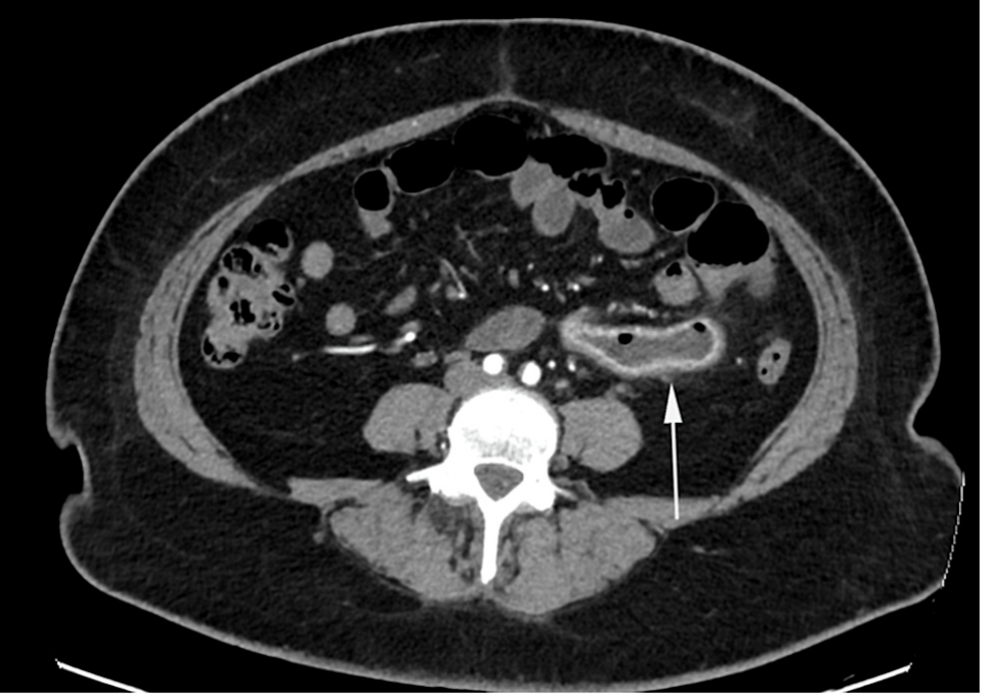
Axial reformatted, post intravenous contrast computed tomography images in the arterial phase White arrow demonstrates mild circumferential mural thickening and hyper-enhancement in a short segment of the jejunum.
